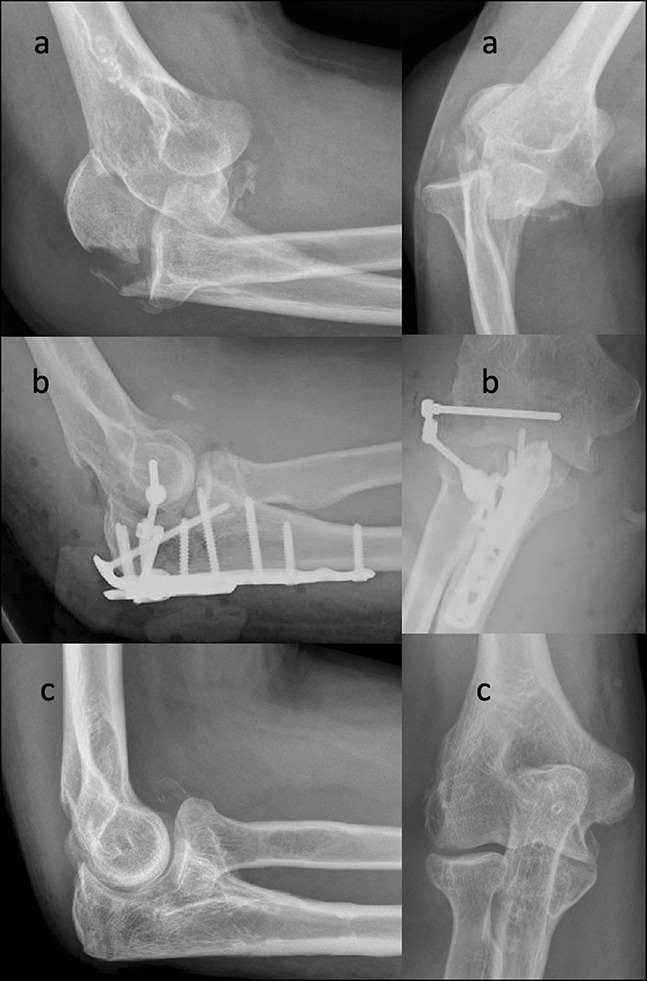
Case 2: Fluoroscopy images demonstrating transolecranon fracture-dislocation (A). Radiographs demonstrating surgical fixation using a proximal ulna plate and internal joint stabilizer (B). Three-year follow-up radiographs demonstrating that the elbow was stable and the patient had satisfactory function (C).
